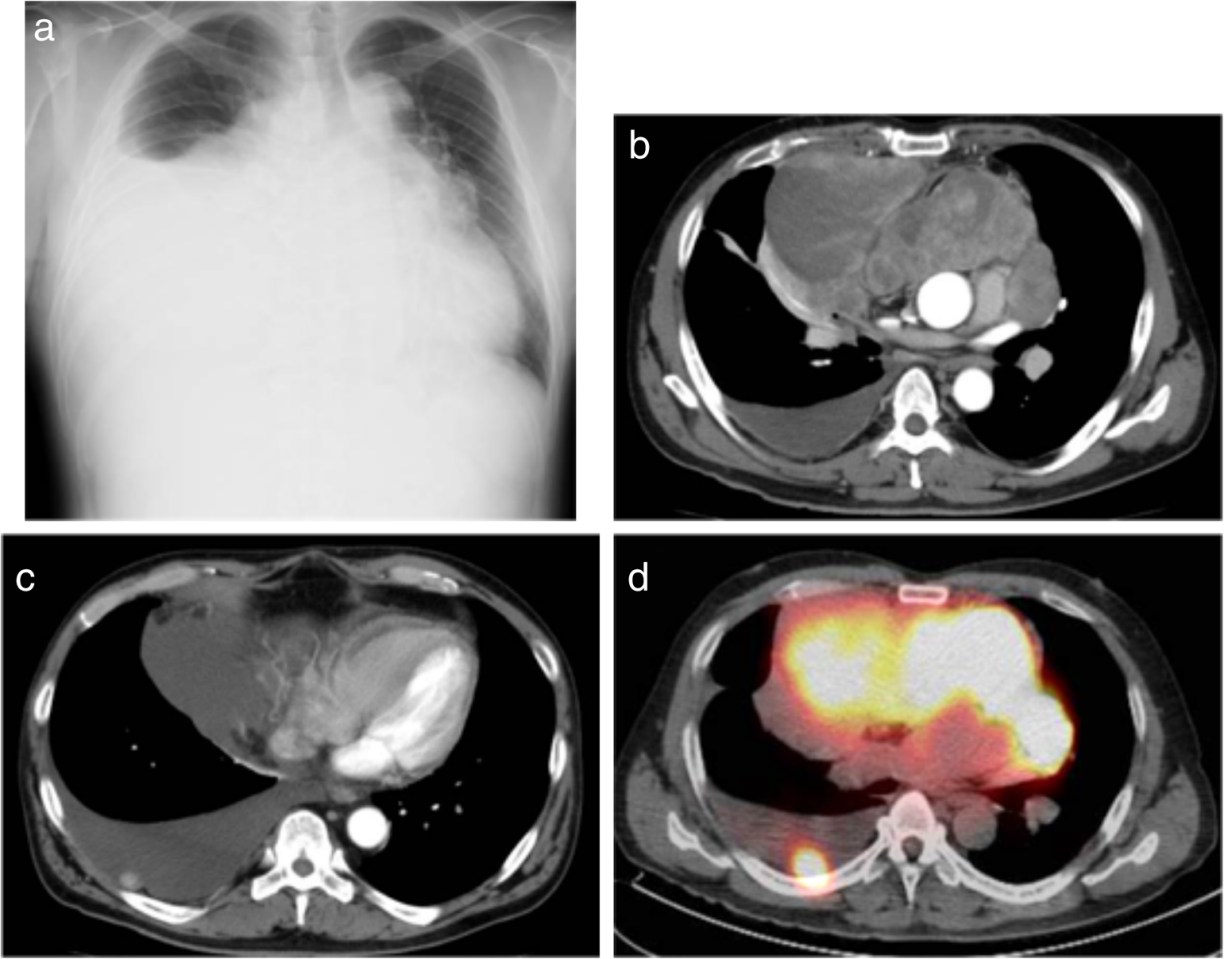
Images from the previous hospital. (a) Chest X‐ray showed an extensive abnormal shadow in the right lower lung field and cardiac expansion. (b, c) Chest computed tomography (CT) revealed moderate right pleural effusion, nodules in the pleura, and a huge tumor at the anterior mediastinum. (d) Positron emission tomography CT detected abnormal high uptake of fluorodeoxyglucose in the mediastinal tumor and nodules in the pleura
